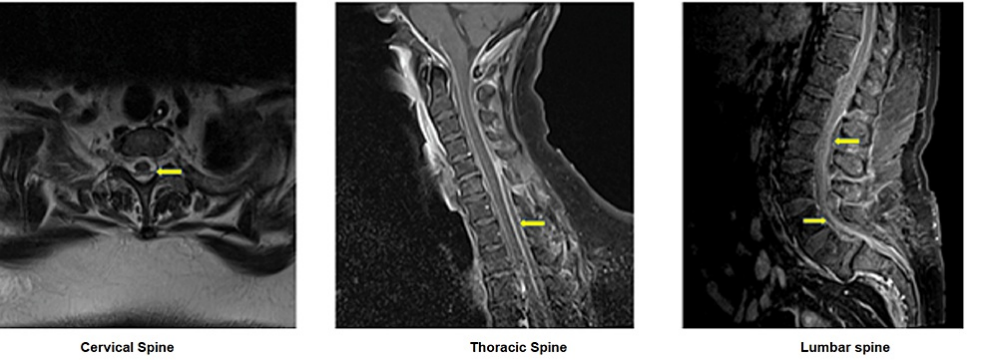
Impression of the cervical, thoracic, and lumbar spine MRI with contrast; yellow arrows show epidural abscess collections around the cervical, thoracic, and lumbar areas, respectively.
